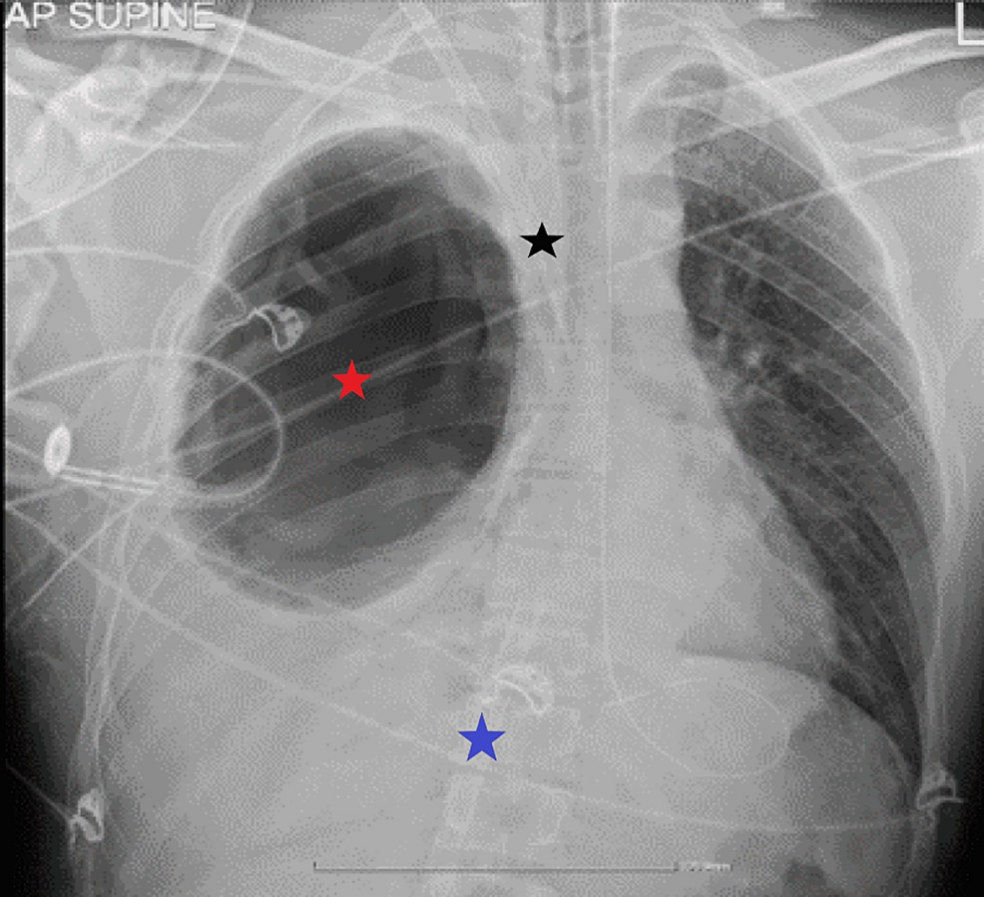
Chest X-ray after ECMO catheter placement Black star: Right subclavian vein ECMO catheter; Blue star: Right femoral vein ECMO catheter; Red star: Echinococcus cyst ECMO: extracorporeal membrane oxygenation
